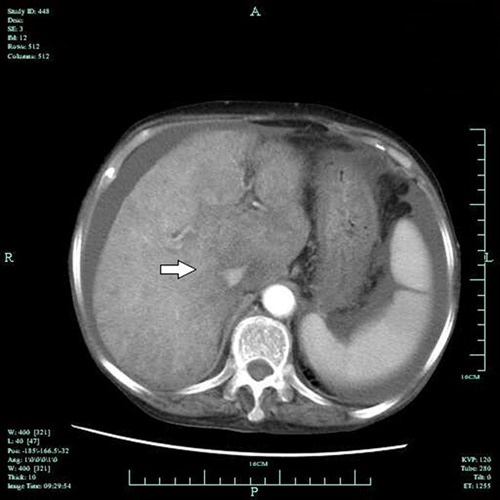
Unenhanced CT image of the inferior vena cava in the liver section of a
